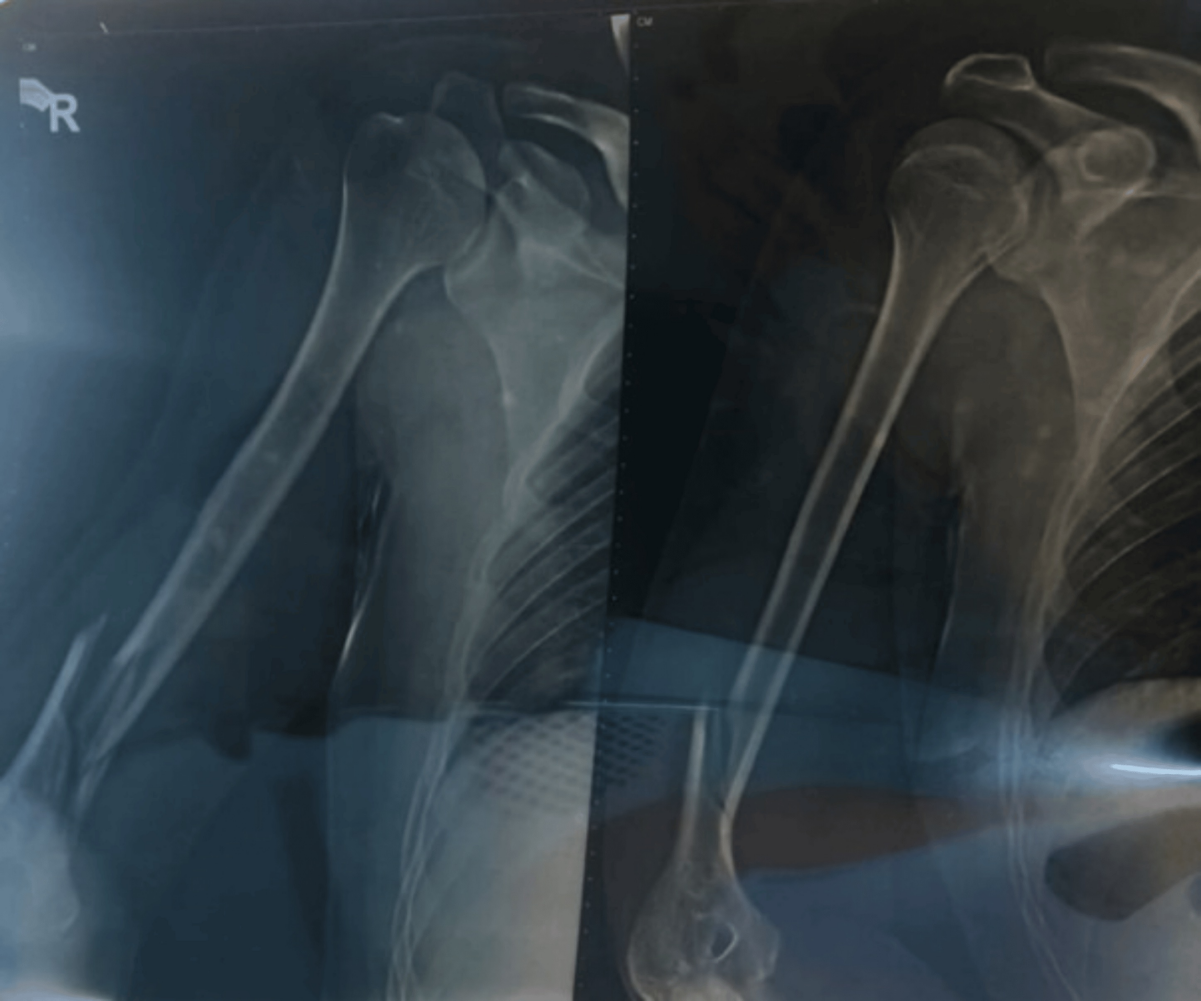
Radiographic image depicting a fracture of the right humerus.
